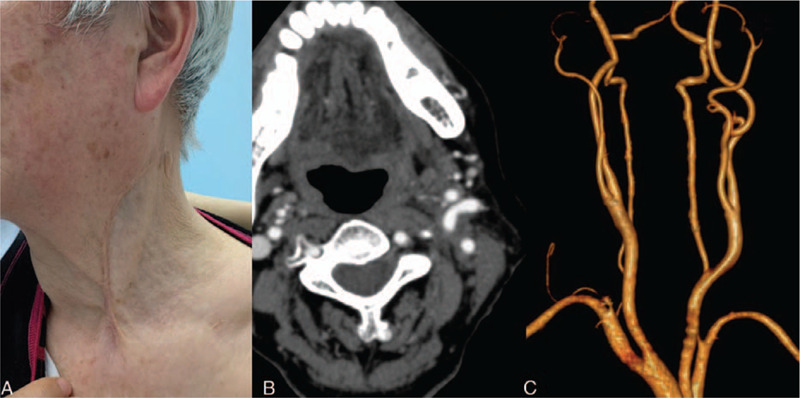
Two-year follow-up of this patient. (A) Appearance of the front view of the neck. (B) Axial view of computed tomography angiography (CTA) of previous operative region. (C) CTA showed carotid arteries were patent and distorted.
